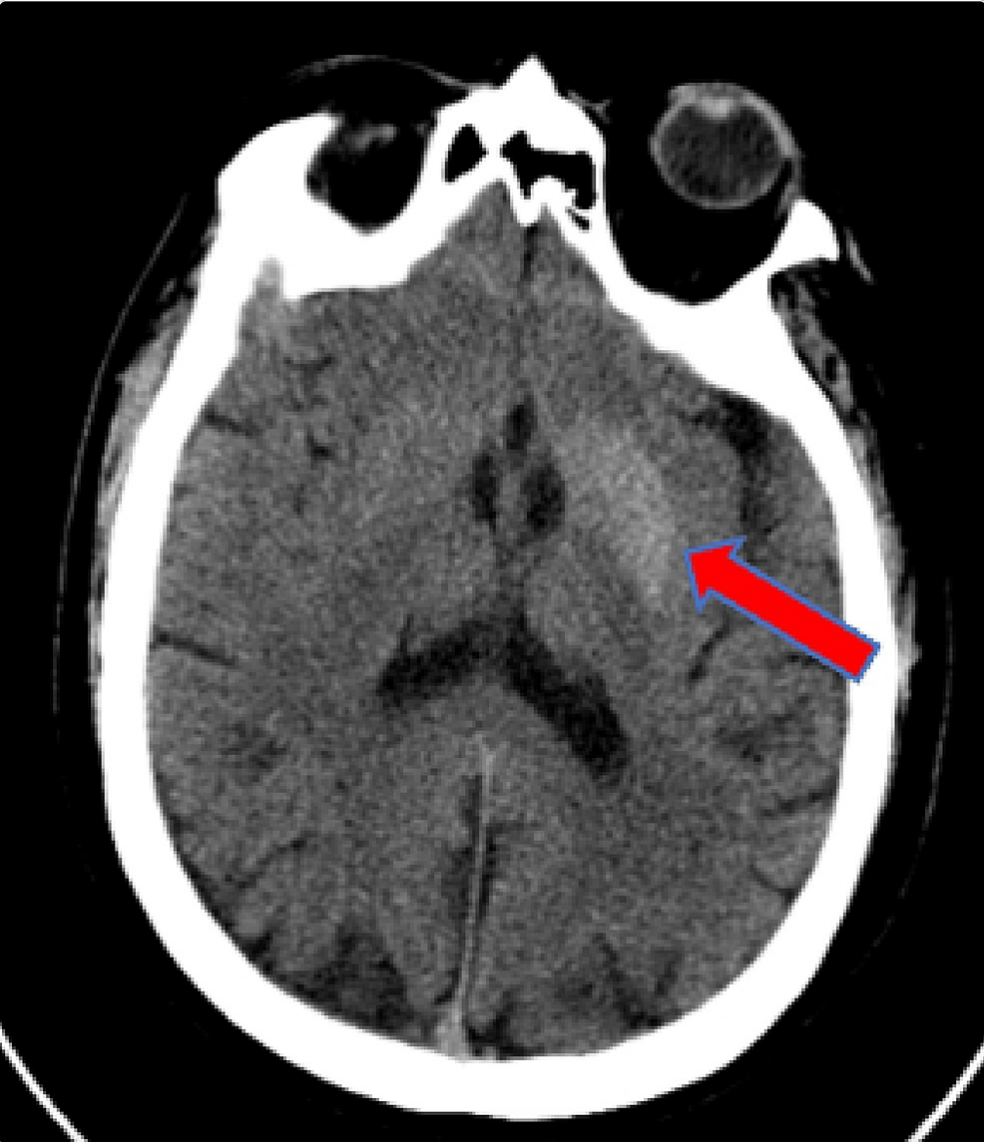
Head CT without contrast Hypodensity in the left putamen and globus pallidus (arrow) CT: computed tomography
